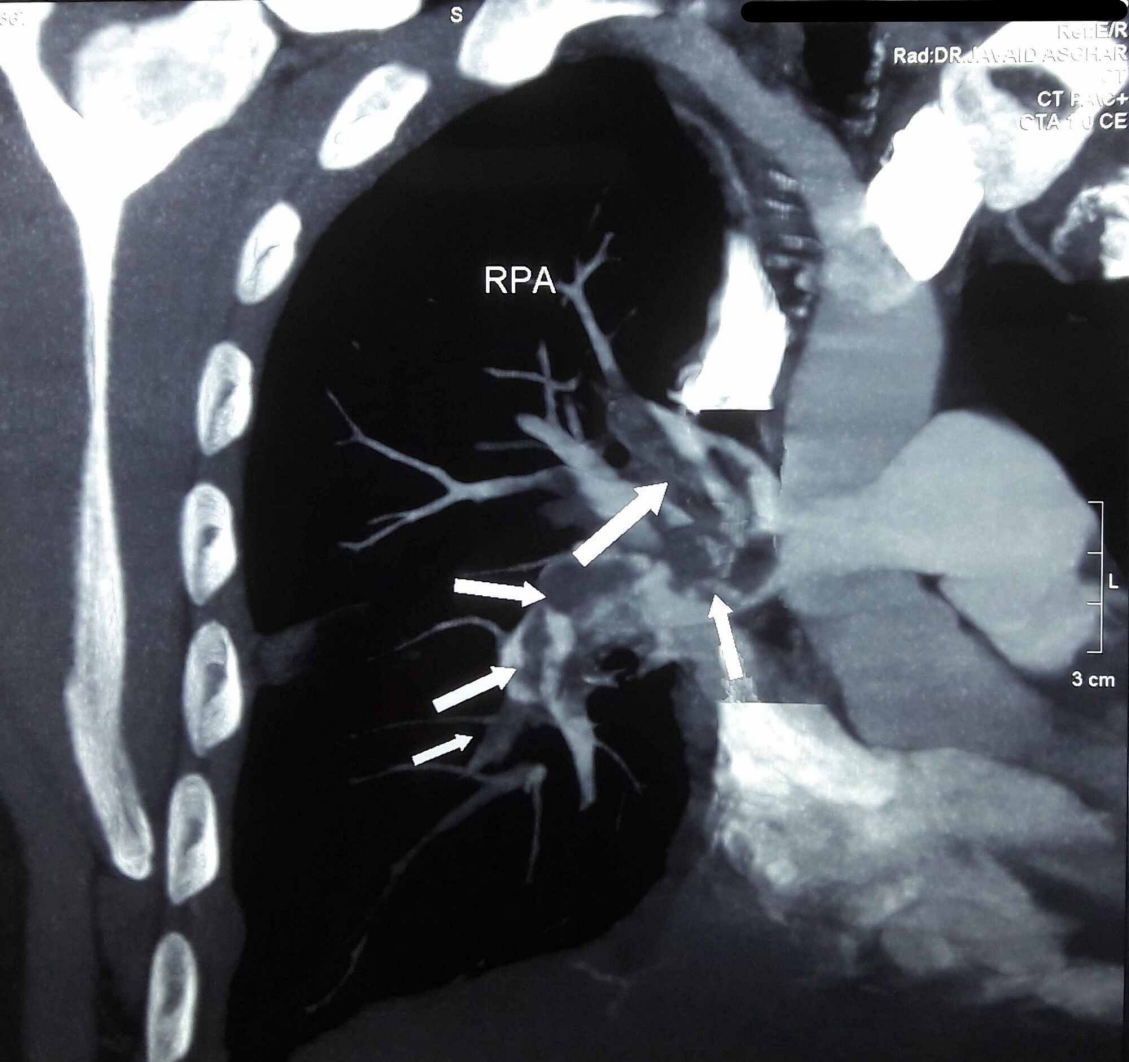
Computed tomographic pulmonary angiography. RPA - right pulmonary artery - occlusive pulmonary thromboembolus involving right pulmonary artery and subsequent branches.
